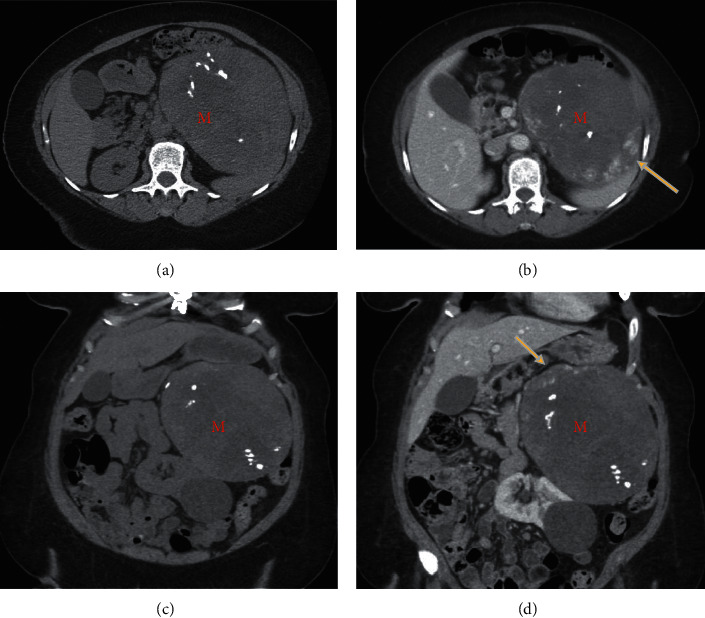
Pre- and postcontrast CT scan of the abdomen: large heterogeneous solid left adrenal mass (M) with internal calcifications and peripheral discontinuous enhancement after contrast (arrows in B and D). *Note*. Significant mass effect of the left kidney which is pushed inferiorly.
